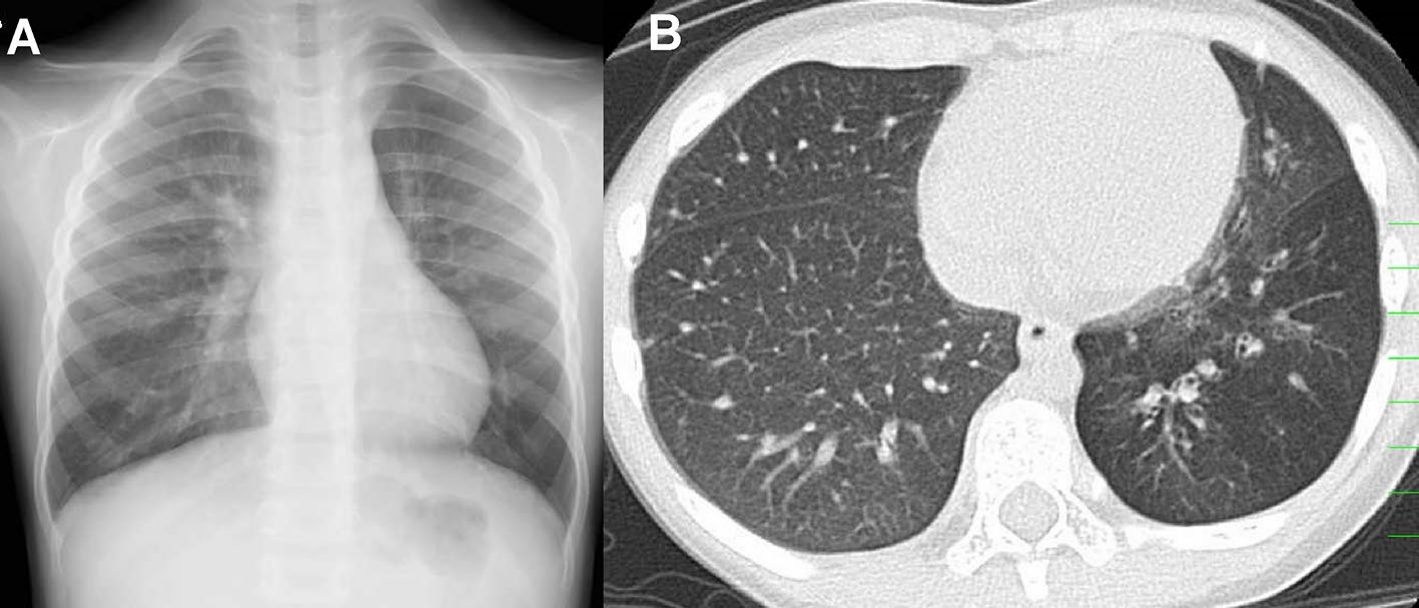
Swyer–James syndrome. A 4-year-old girl with a history of adenovirus pneumonia. A CXR showed unilateral lucent lung with reduced volume on the left side. B CT revealed generalized hyperlucency with attenuated vascular shadows and bronchial wall thickening of the left lung
